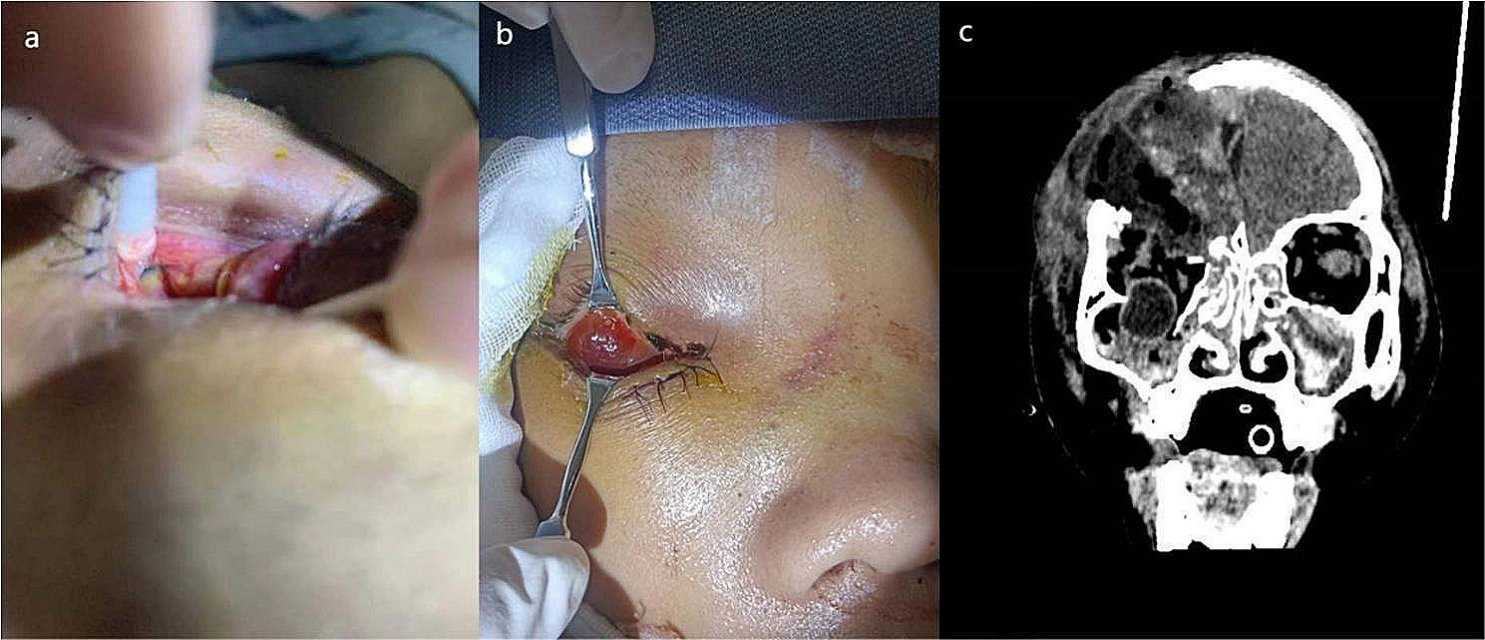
Right orbital cavity without eyeball on physical examination. (a). The dislocated eyeball could be observed from the patient’s head downwards. (b). Only conjunctival oedema was observed under direct vision. (c). Computed tomography showed extensive fractures of the orbital floor and eyeball displacement into the maxillary sinus
